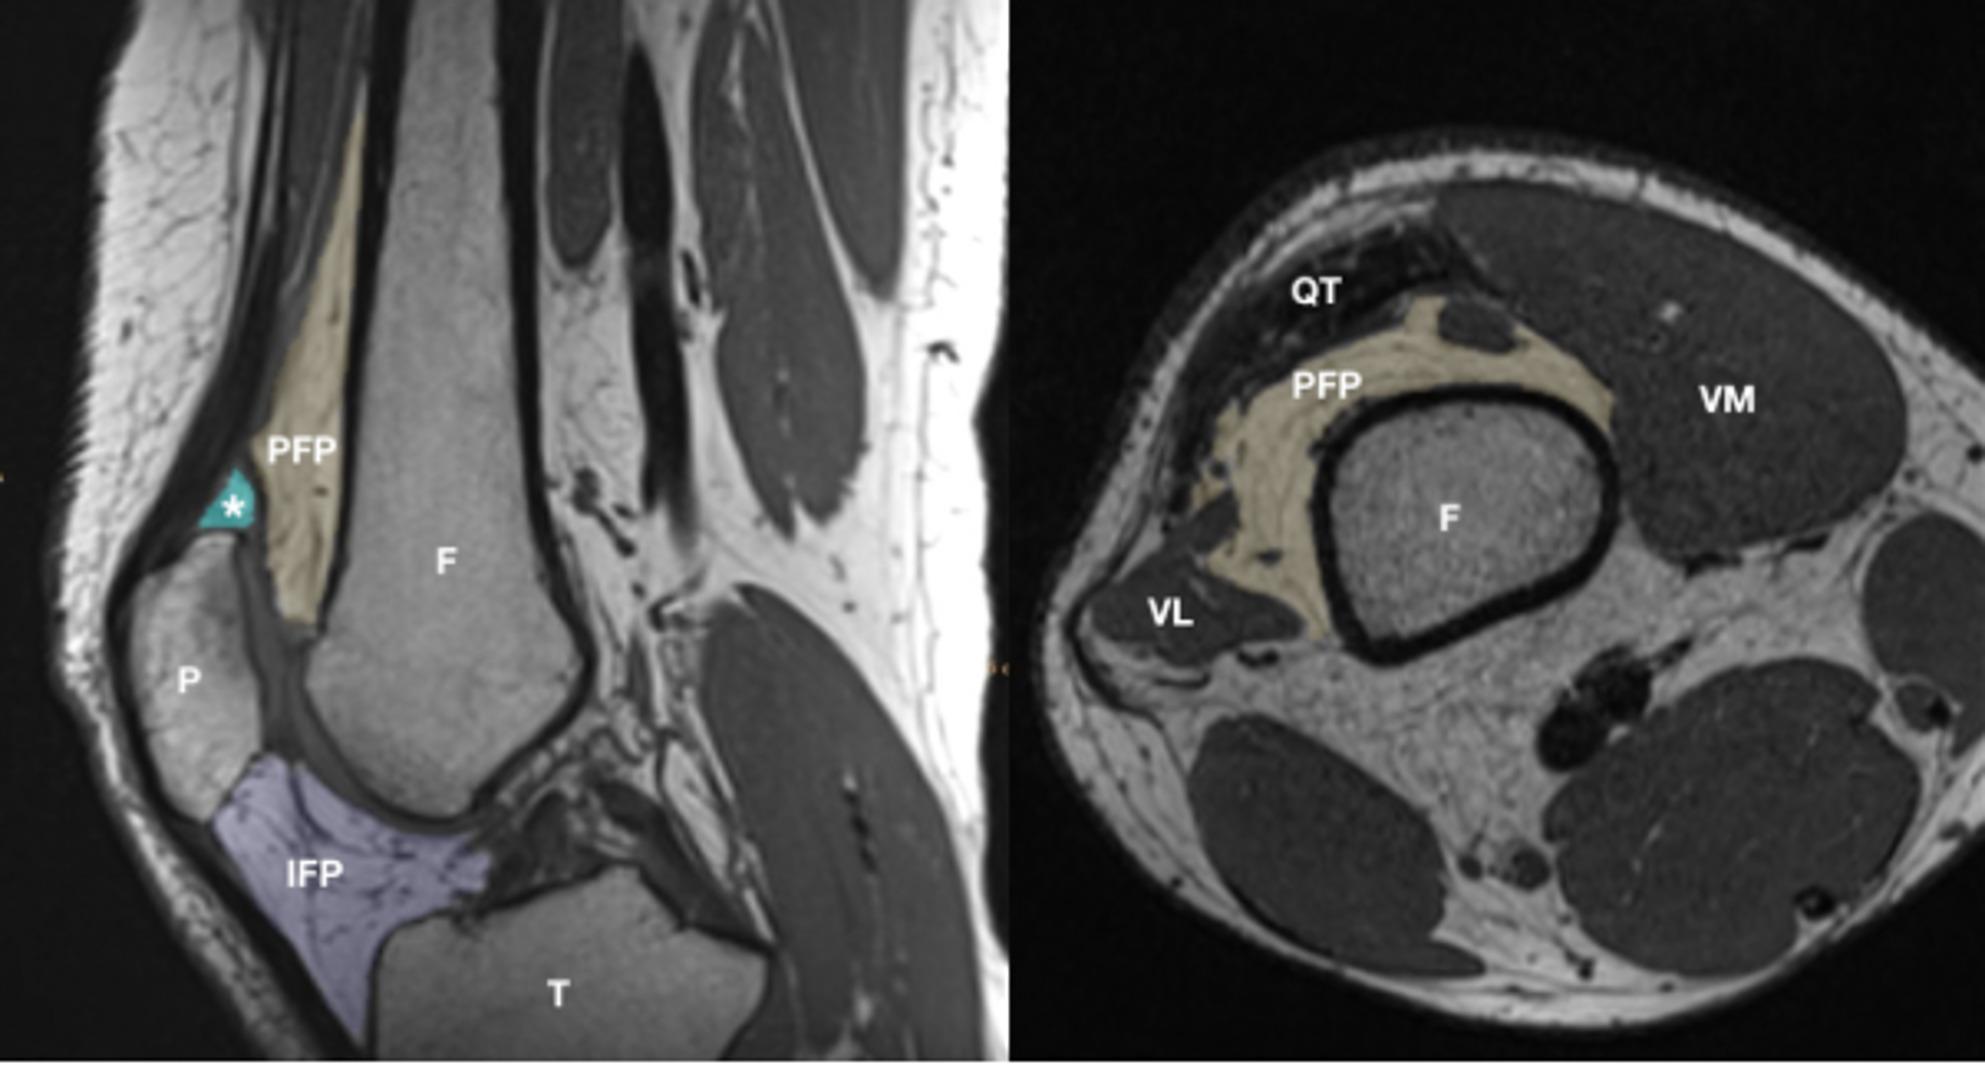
T1-weighted MRI scan in the sagittal and axial plane of the prefemoral fat pad (PFP, yellow). The infrapatellar fat pad (IFP, purple) and the suprapatellar fat pad (SFP *, blue). PFP Boundaries are defined as the femur (F) dorsally, the quadriceps tendon (QT) and suprapatellar recess ventrally, the trochlear cartilage distally, the lateral border of the medial vastus muscle (VM) medially and the medial border of the lateral vastus muscle (VL) laterally
